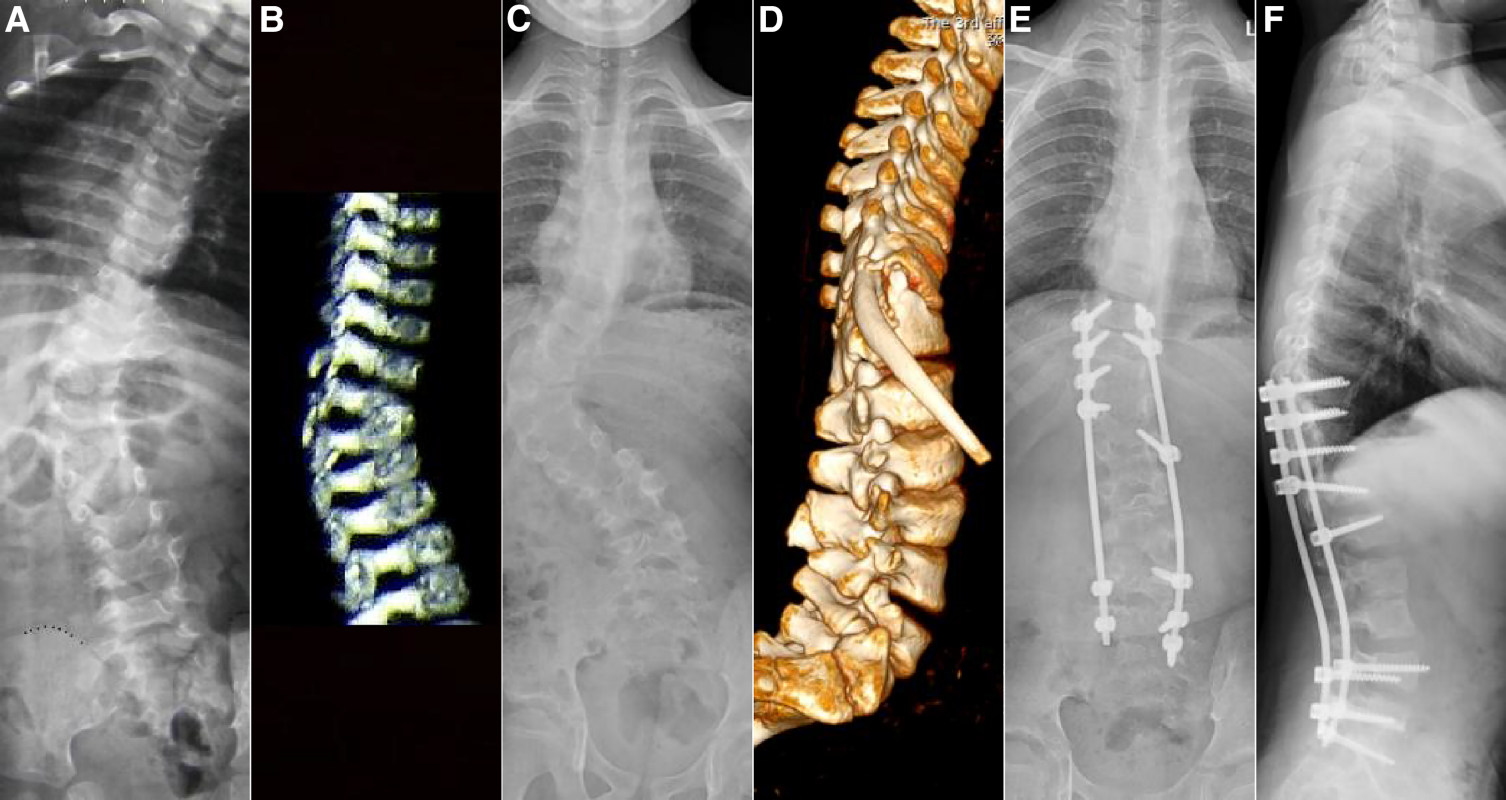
Case 15, female, 11 months old. Preoperative orthogonal x-ray (A) and lateral computer tomography (B) showing the right hemivertebrae of T11. Intraoperative hemivertebra resection was performed *via* the posterior approach. After 9 years of follow-up, orthogonal x-ray (C) and orthogonal computer tomography (D) showed local pseudoarticular formation and worsening deformity, and a second internal fixation revision surgery was performed. Post-revision orthogonal (E) and lateral (F) x-ray showed good correction of the deformity in the coronal and sagittal planes.
